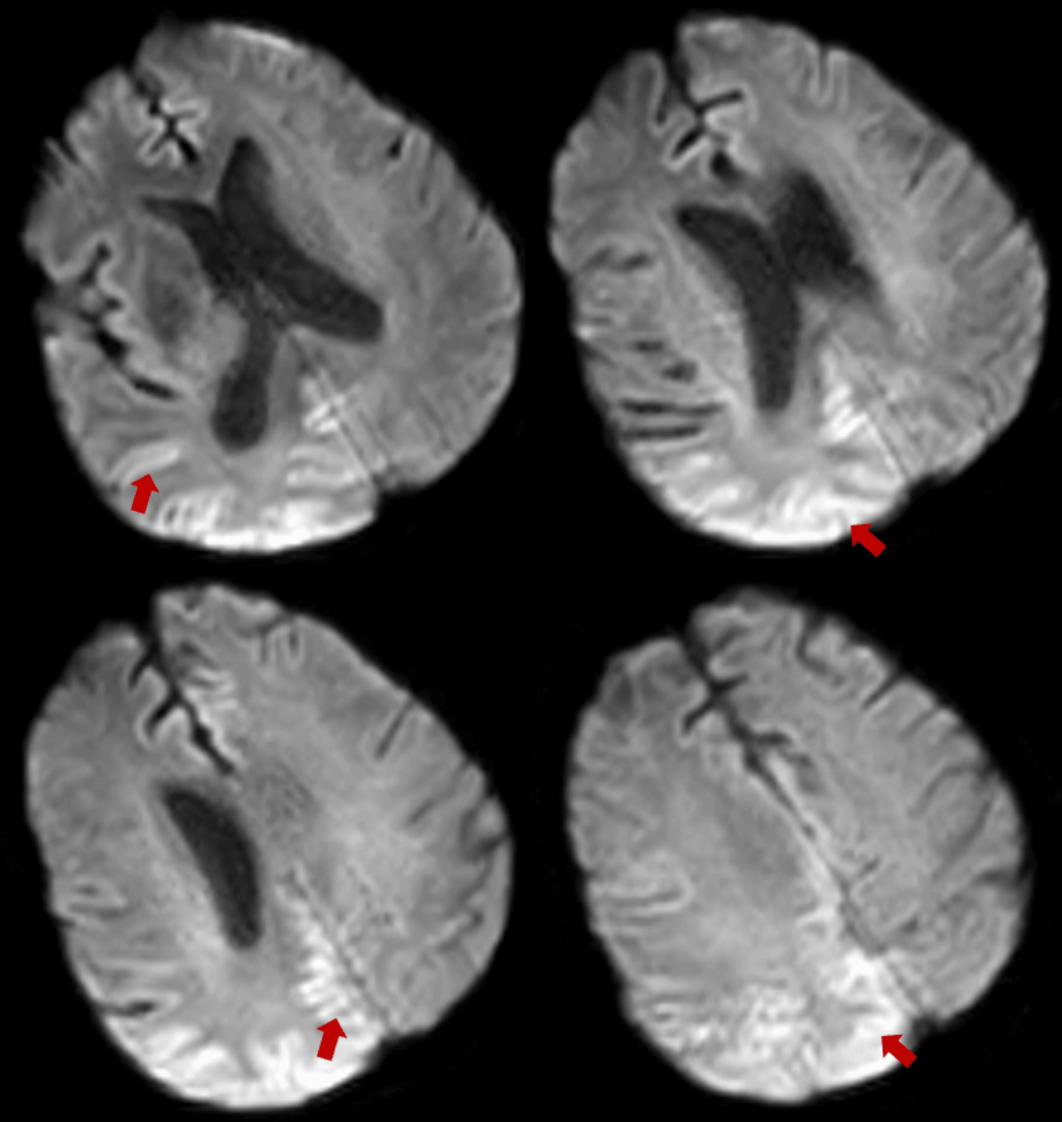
Final MRI brain without contrast. Findings shown: DWI axial sequences showing parietooccipital cortical ribboning highlighted by the arrows. There was significant movement artefact during the study.
